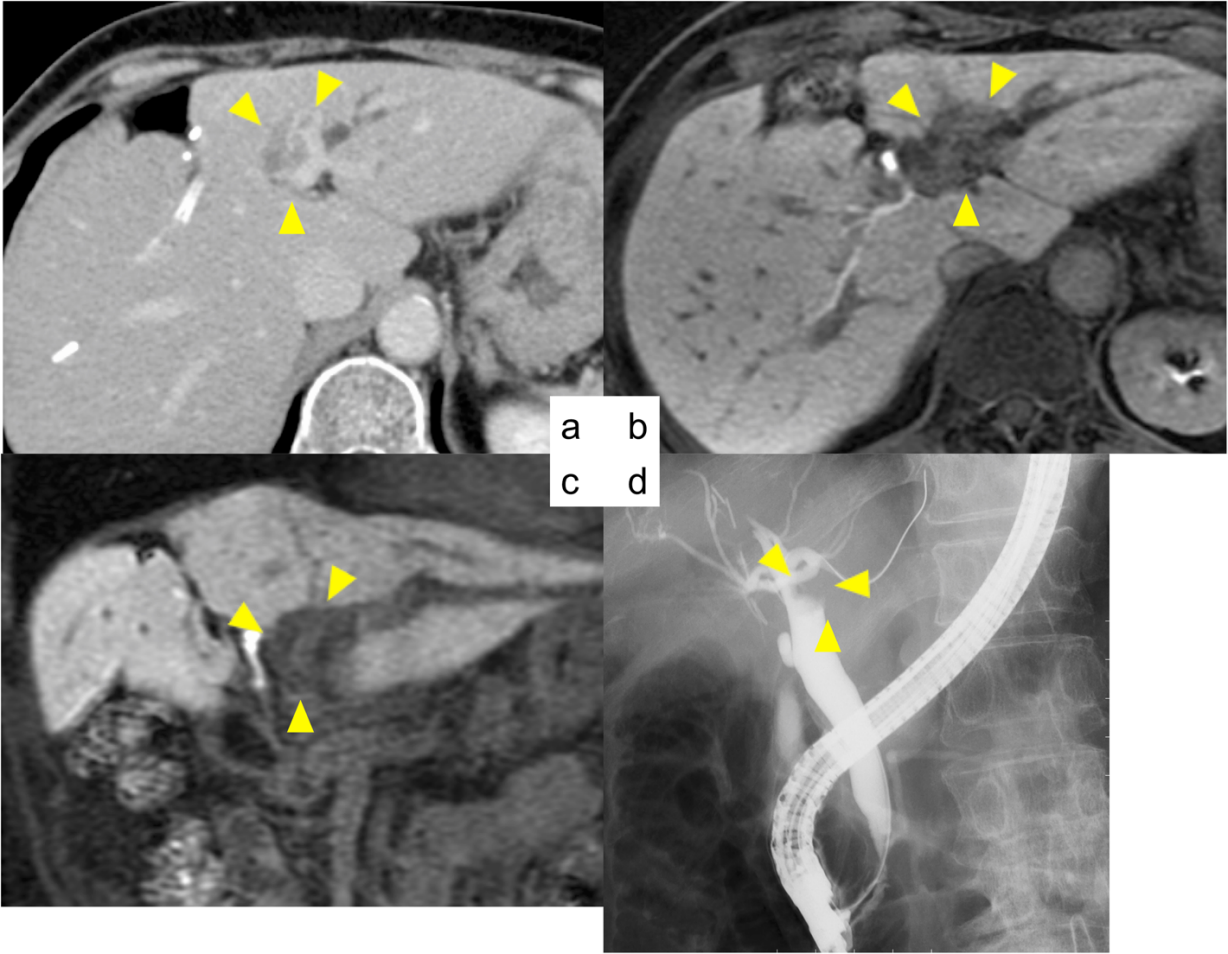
a Contrast-enhanced CT scan revealed dilation of left intrahepatic bile duct (8 mm) and a high-density mass in segments II and III. b, c MRCP also revealed a 20 × 14 mm mass in segments II and III. d ERCP revealed a shadow defect in segments II and III. No other tumor or defect was detected
